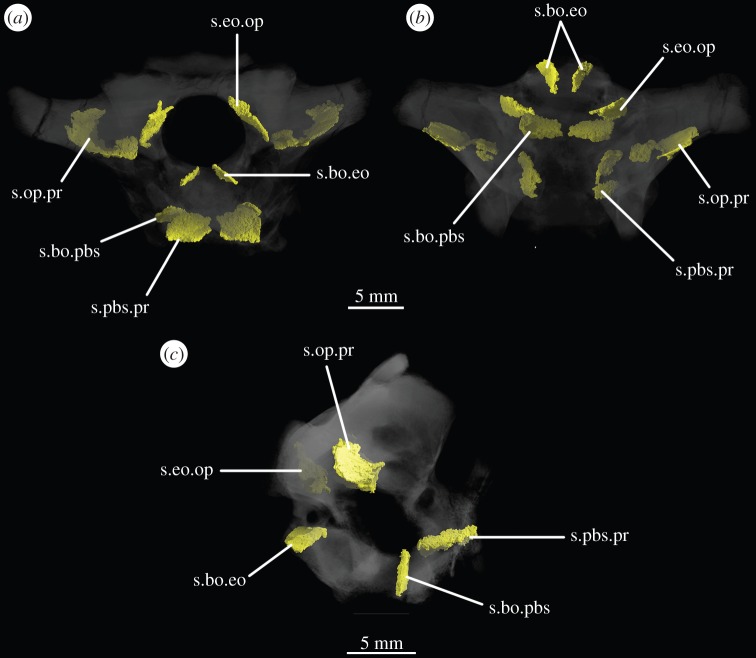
CT reconstructions of the braincase of SAM-PK-7696 showing sutures in (*a*) anterior, (*b*) ventral and (*c*) right lateral views. Bones of the braincase have been rendered transparent for better visualization of the suture lines. For abbreviations, see table 1.
